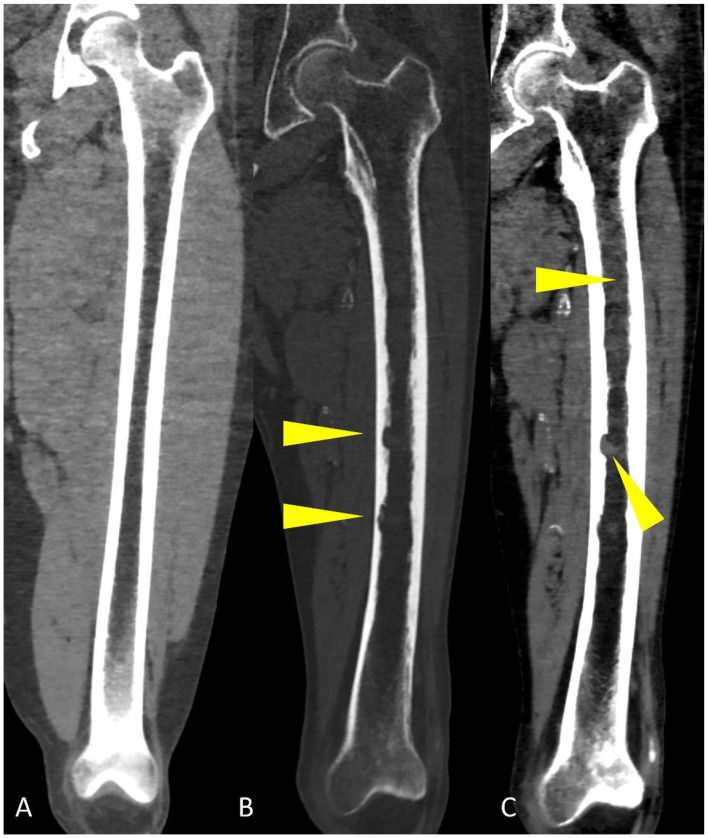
Coronal CT reconstruction images of the femoral bone showing: (A) regular aspect of bone marrow without osteolytic lesions, endosteal scalloping and pathological deposits; (B) “bone” algorithm reconstruction, used to evaluate osteolytic lesions and endosteal scalloping (yellow arrowheads); (C) “soft tissue” algorithm reconstruction used to assess focal and diffuse hyperdense deposits in the medullary cavities of long bones (yellow arrowheads).
